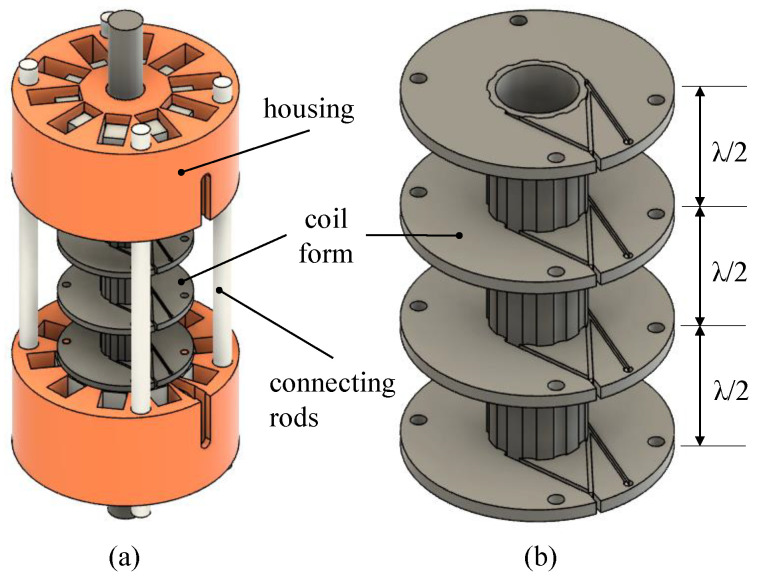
Assembly of the EMAT sensor showing the housing that encloses the components, connecting rods that maintain alignment, and the coil form (a). Detailed view of the coil form, highlighting the three segments, each corresponding to half the wavelength (λ/2) of the ultrasonic waves (b).
